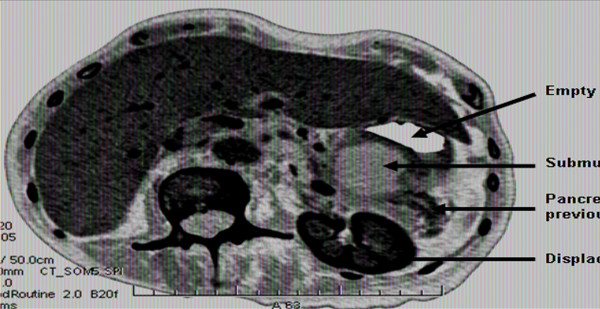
CT abdomen showing cystic recurrent GIST located in the region of the pancreatic tail.
